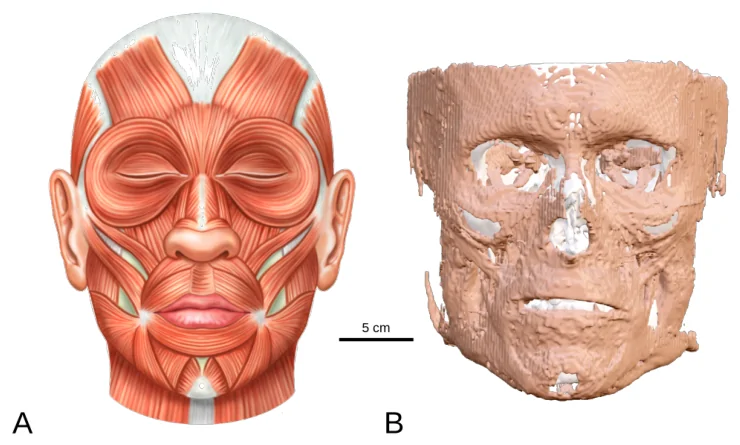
Comparison of the typical frontal view of the facial muscles in anatomy textbooks (A) and the muscles segmented, shown as a 3D reconstruction of the MRI dataset from male donor #12 (B). The textbook does not clearly reflect the complexity and interdependence of the facial musculature.
